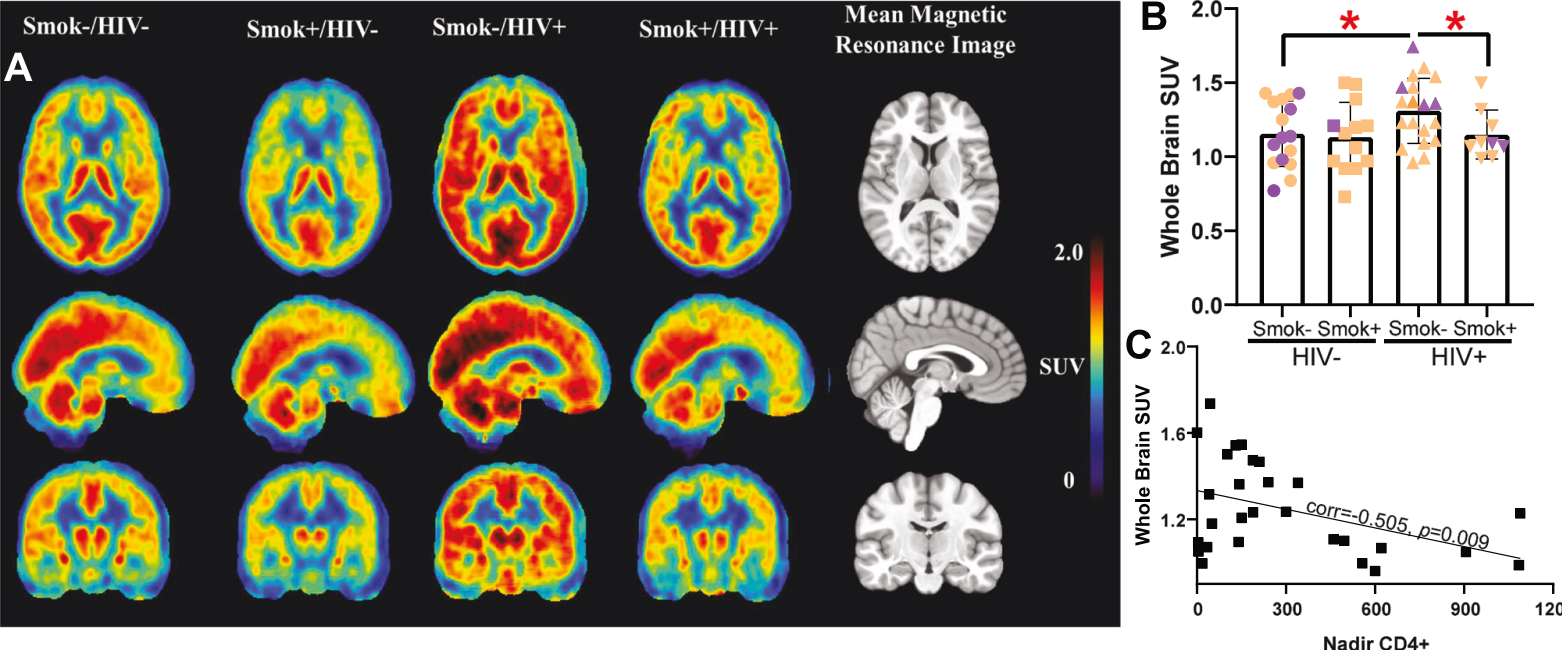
PLWH that smoke cigarettes have reduced levels of the marker for neuroinflammation relative to their non-smoking counterparts. Mean PET/CT images from participants who do not have HIV (HIV-) vs. those that do (HIV+), and those that smoke cigarettes (Smok+) vs. those that do not (Smok-). As can be seen, the highest (brightest red) levels of the marker for neuroinflammation were seen in HIV+Smok- participants, while PLWH who smoke had reduced levels (HIV+Smok+; A). These levels were quantified and found to be significant (B). Levels of neuroinflammation negatively correlated with nadir CD4+ levels (C). *=*p* < 0.05 as indicated.
